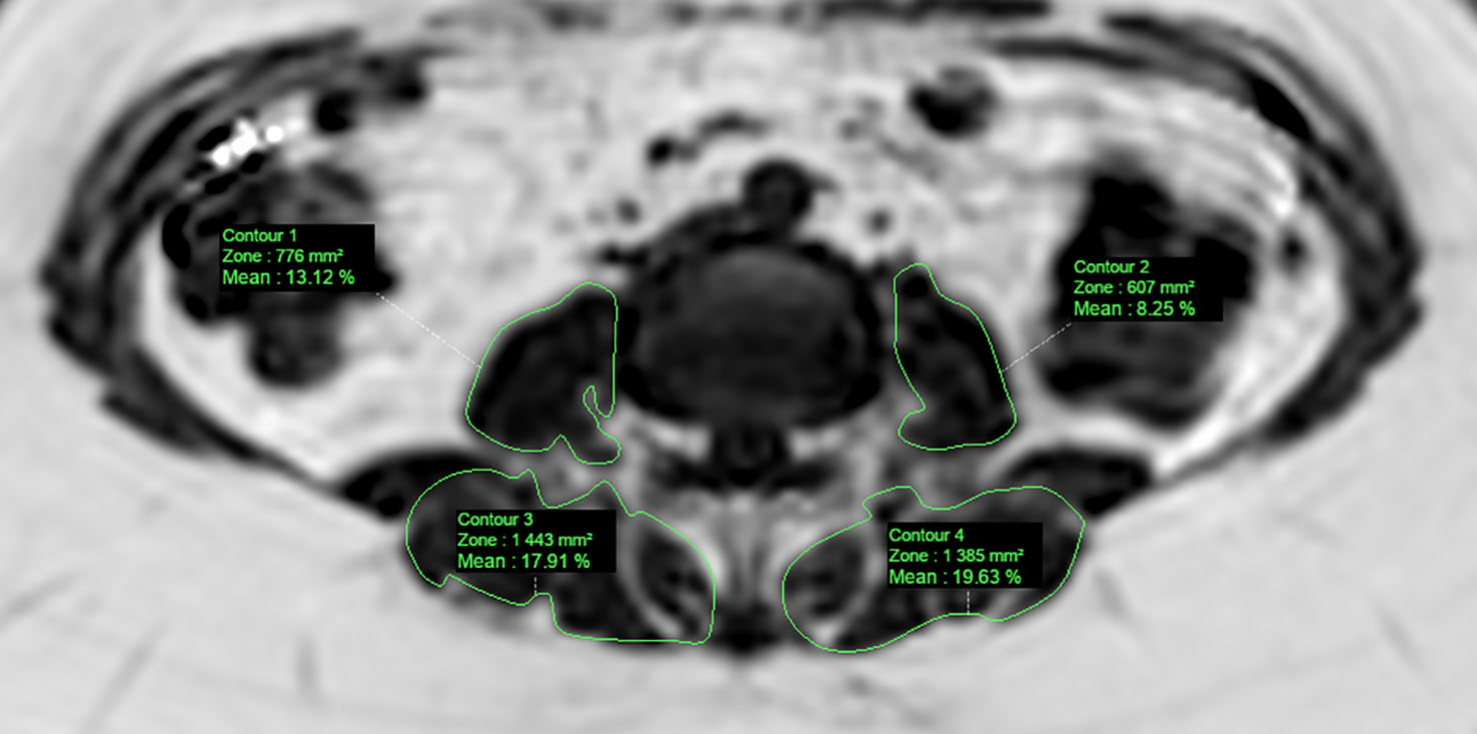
PDFF map of the paravertebral muscles computed from a T1-weighted multi-echo gradient echo sequence (mDixon-Quant) acquired in an axial oblique plane (L3-L4 level), from a 58-year old case. Manually segmented ROI (*green*) was placed in the psoas and paravertebral (multifidus+erector spinae) muscles.
